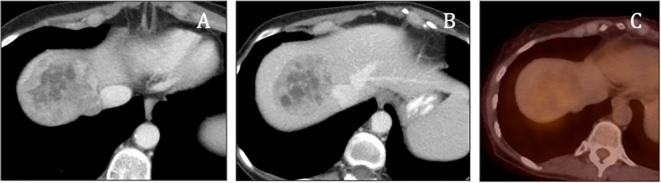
PET/CT demonstrated minimal activity in the lesion (A-C).
